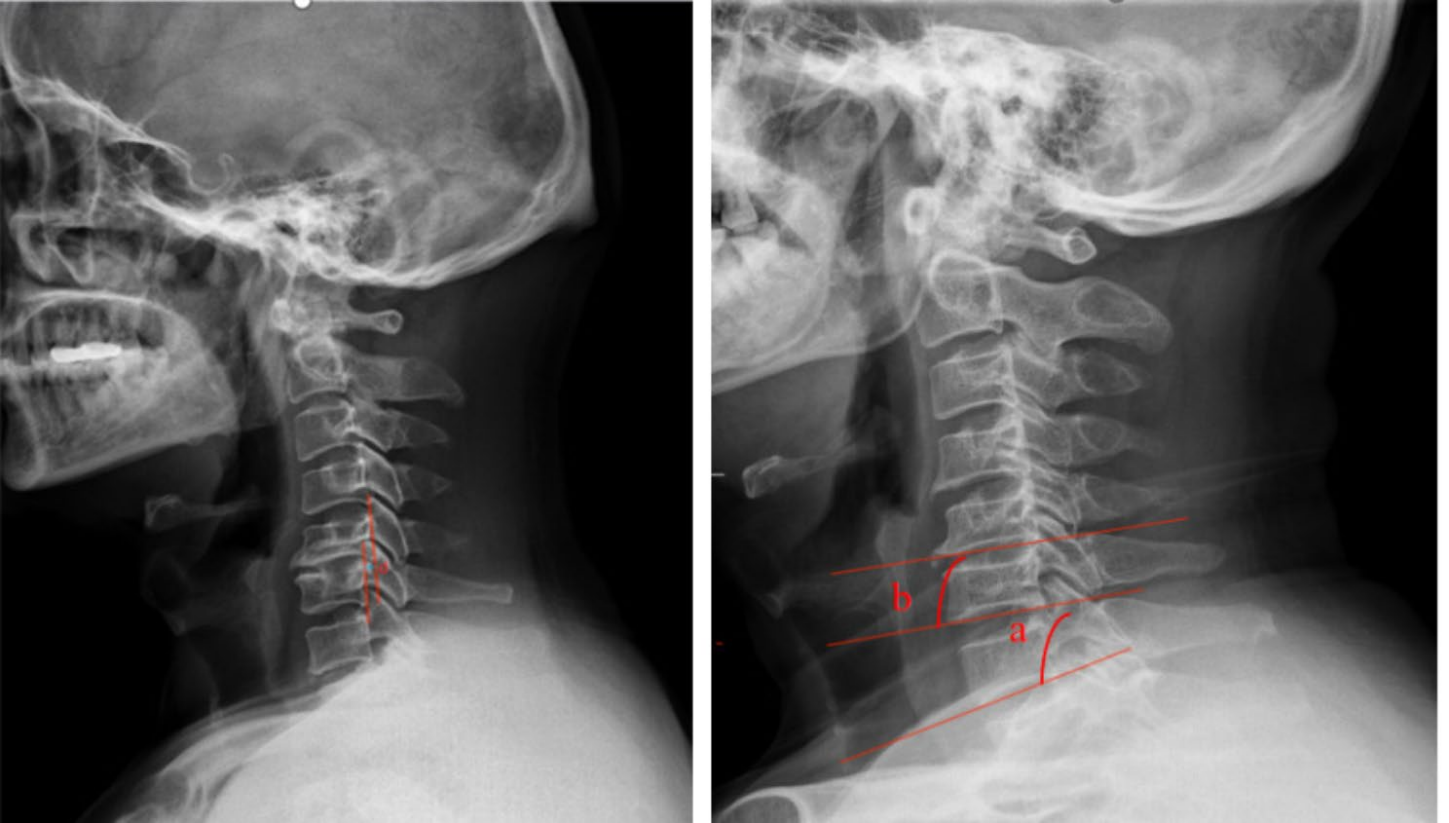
Translational instability is defined as more than 3.5 mm horizontal displacement of one vertebra in relation to an adjacent vertebra measured on lateral roentgenograms (d ≥ 3.5 mm, left). Rotational instability is defined as more than 11 degrees rotational difference from that of either adjacent vertebra (a–b ≥ 11°, right)
